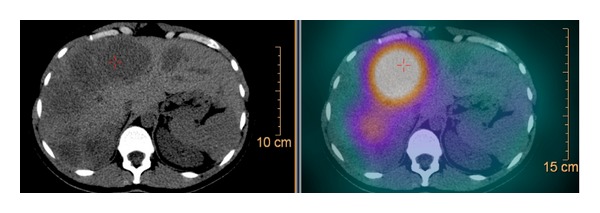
Octreoscan SPECT/CT prior to radiolabeled somatostatin analog lutetium (^177^LU) administration.
